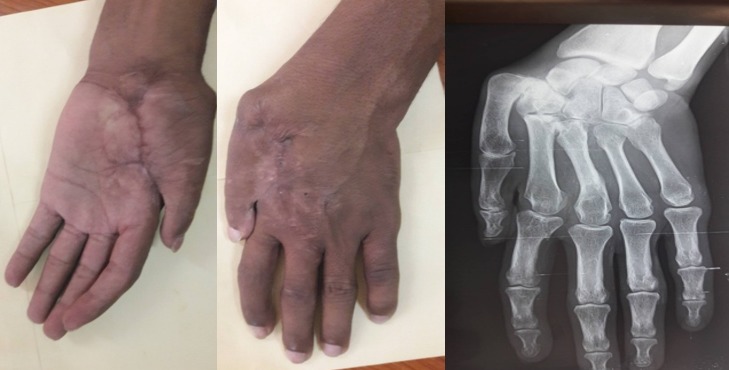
Aspect clinique initial + radiographie initiale
